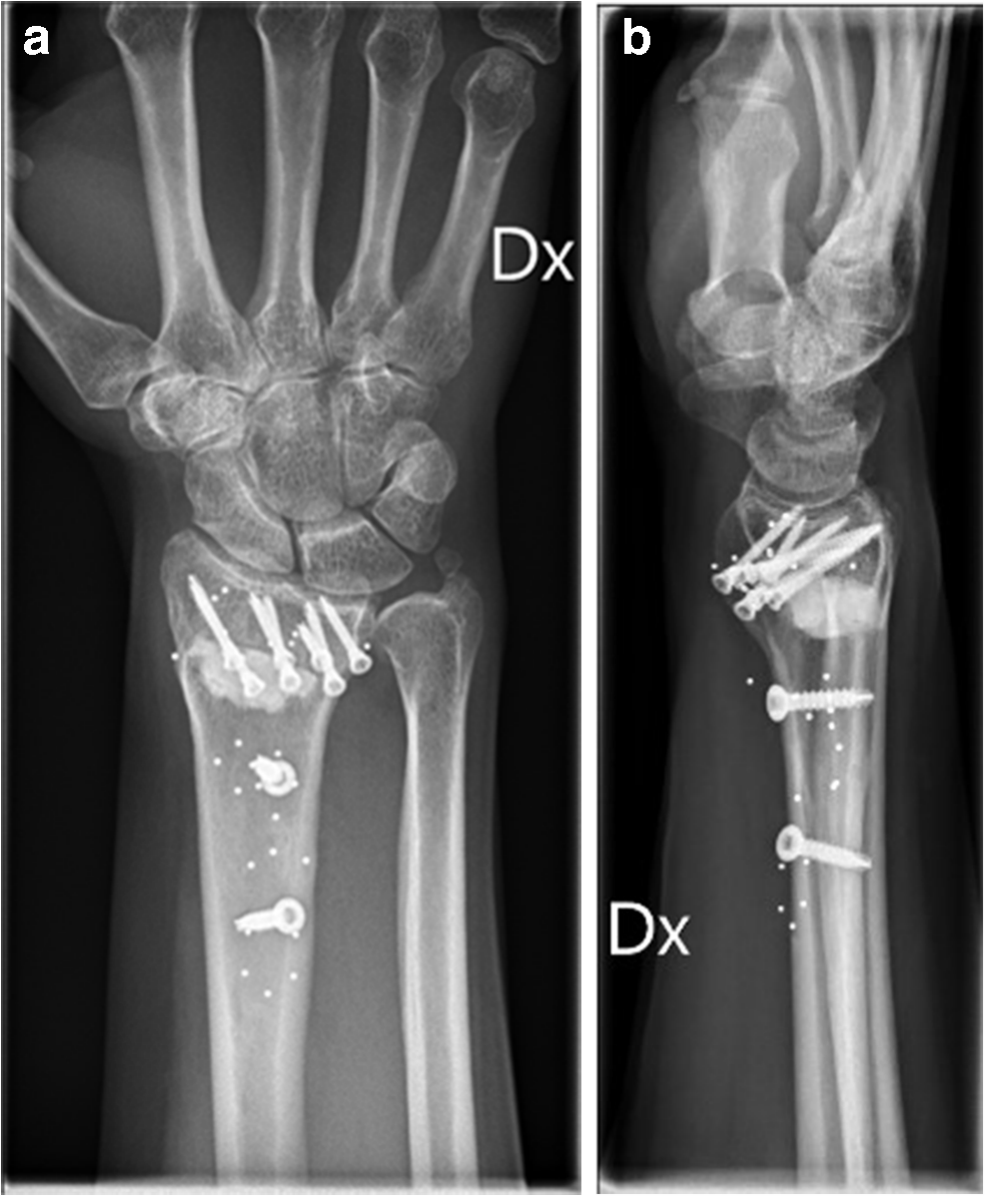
Radiographs at 12 months control after osteotomy using bone substitute. a AP view. b Sagittal view
